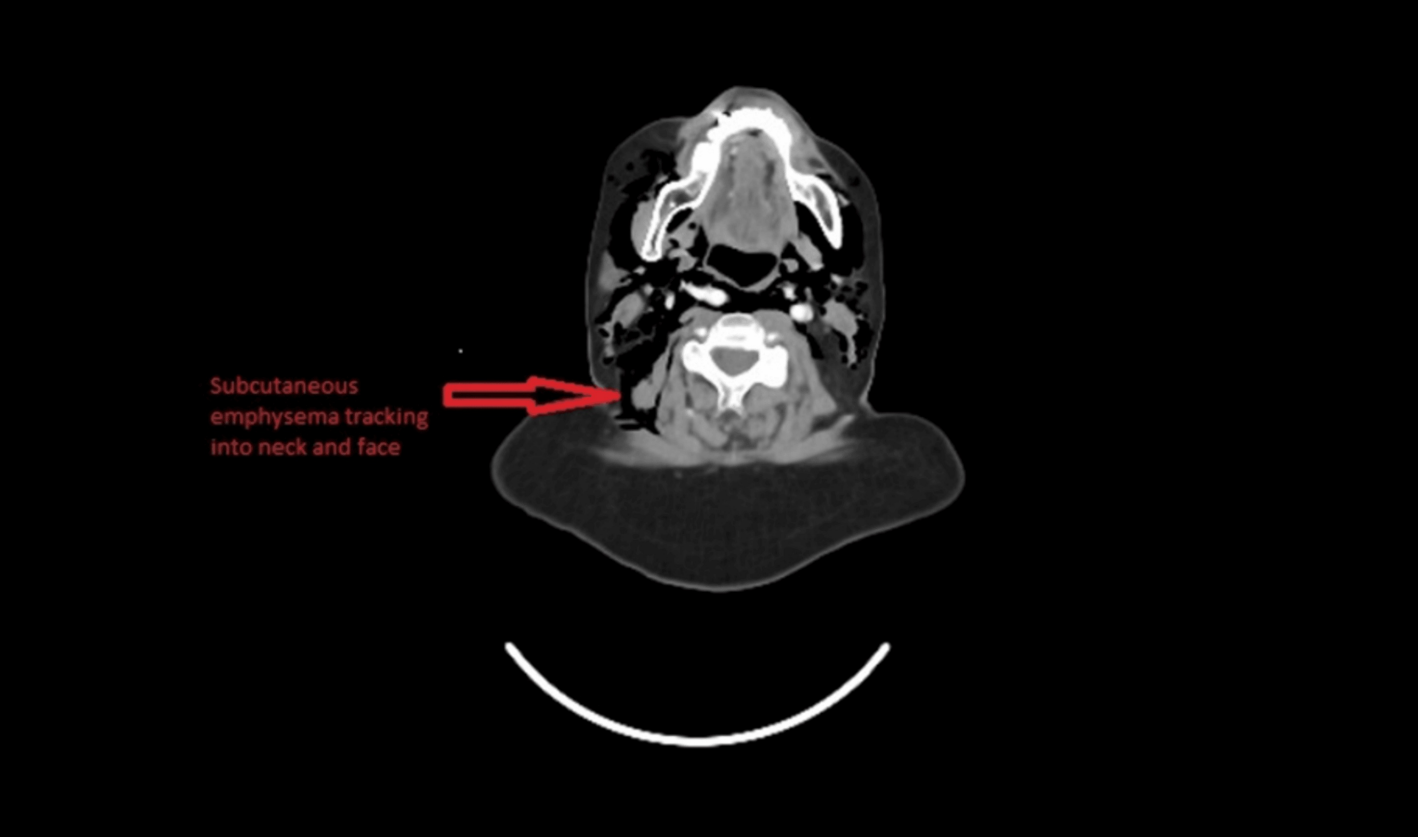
CT neck and chest on 23/09/25. Contrast-enhanced CT of the chest and neck showing subcutaneous emphysema tracking into the neck and face. There is no evidence of superior vena cava obstruction, mass expansion, or port-a-cath leakage, confirming a non-compressive etiology for the swelling.
